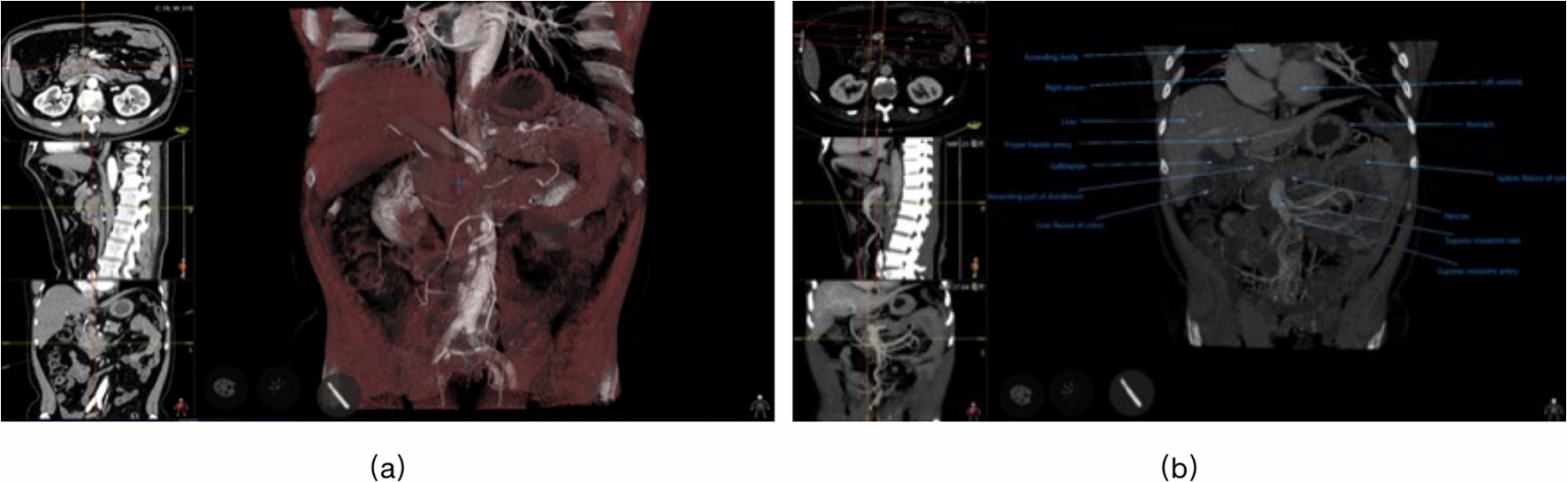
Tumor and adjacent arteries in the a artery-organ preset and b vein-organ preset. Location of the pancreatic tumor was marked on the coronal, axial, and sagittal CT images with cross cursors, and the blue marker indicates its corresponding location in the 3D reconstructed model
